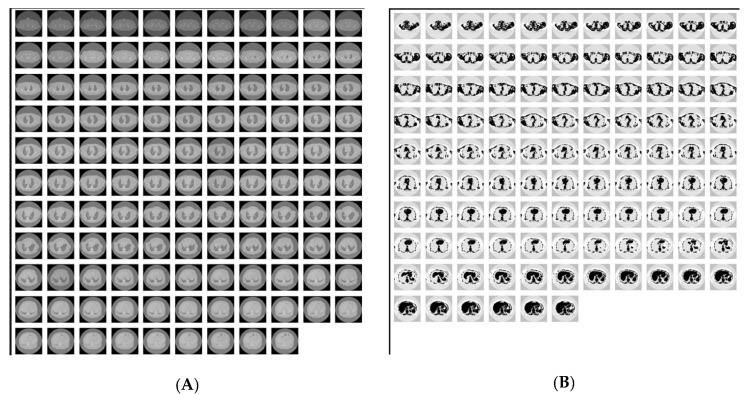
Representative example of a false positive in potential duplicate detection of CT examinations. (A) Shows the total number of comparisons performed, highlighting instances where similar images were detected, categorized by modality. (B) Displays the inter-directory comparison results specifically for the CT modality.
